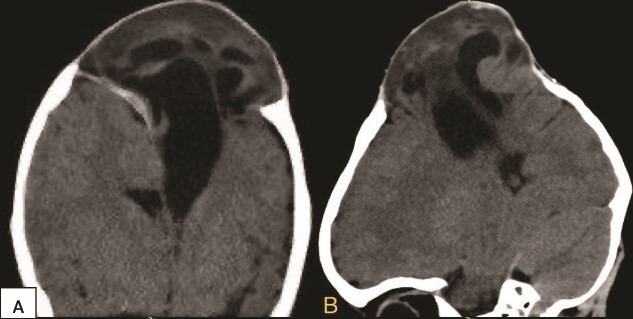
Preoperative coronal (A) and sagittal (B) brain CT scan demonstrating the encephalocele.
